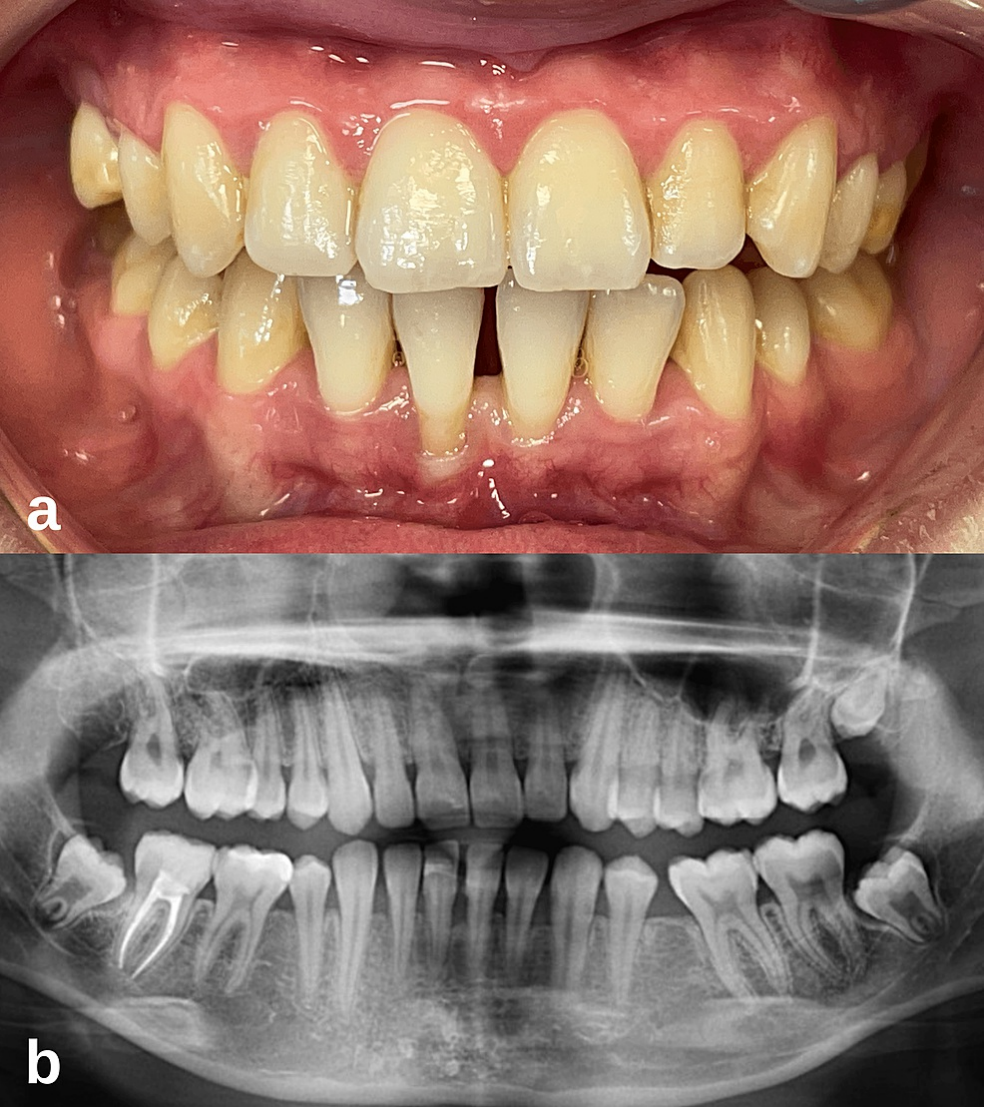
Case 2 initial periodontal examination. (a) Initial clinical intraoral image. (b) Detection of interdental bone resorption in radiographic examination.
